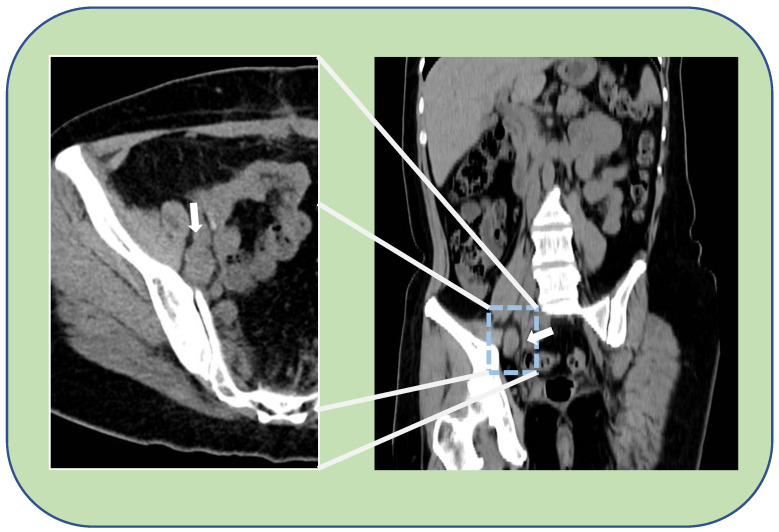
Thick white arrow shows an enlarged, pathologic, lymphnode of about 2 cm in the right obturator region. On the left, a detail of the right half-pelvic cavity on the axial view. On the right, a coronal view of the CT-PET performed during the follow-up.
